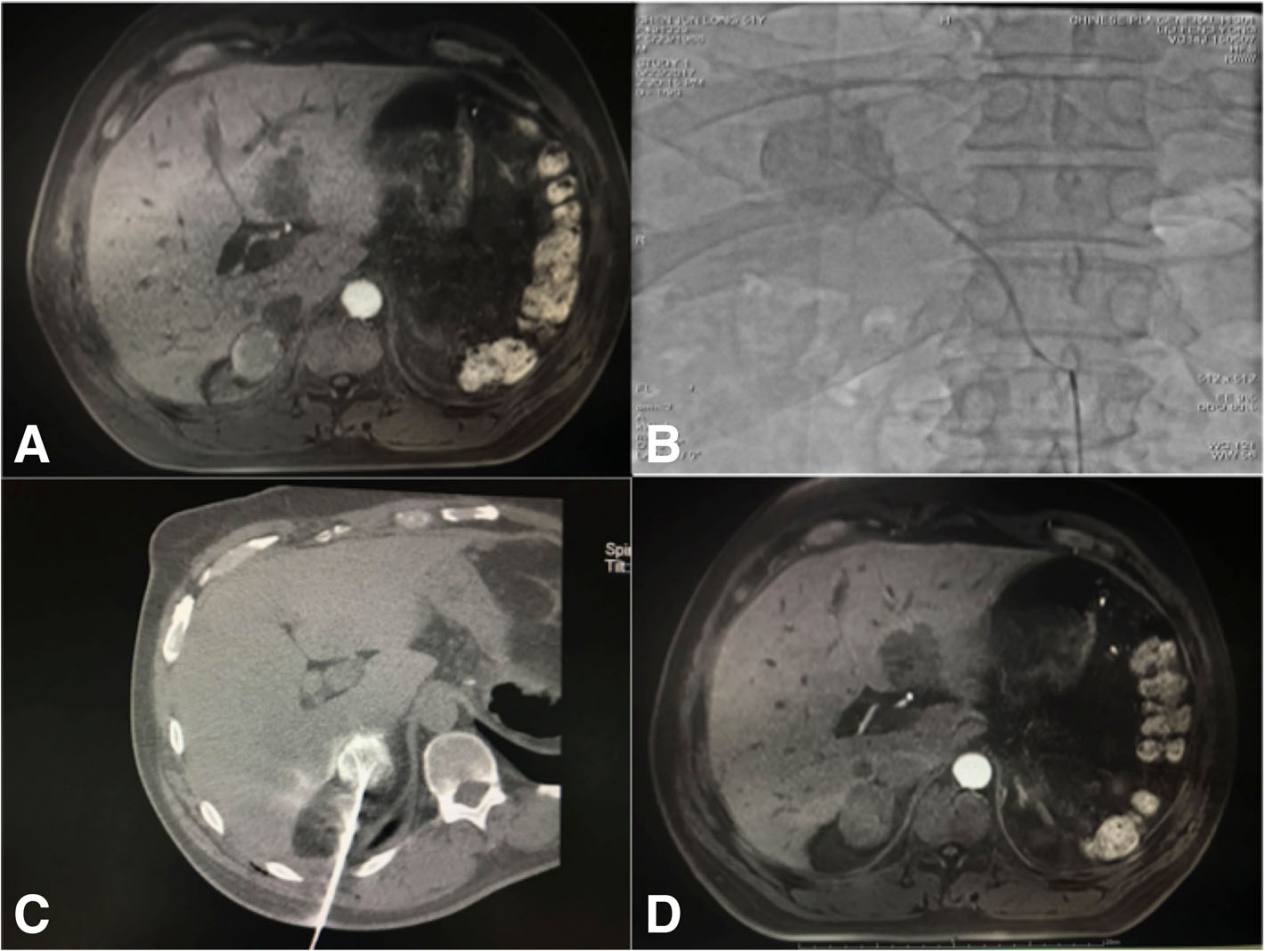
A 52-year-old male patient with right adrenal metastases from HCC who received TACE+RFA. a Enhanced MRI before surgery, indicating adrenal metastases with uneven enhancement. b TACE of the adrenal metastases. c RFA immediately after TACE. d Enhanced MRI 1 month after surgery, indicating no enhancement or coagulation necrosis
